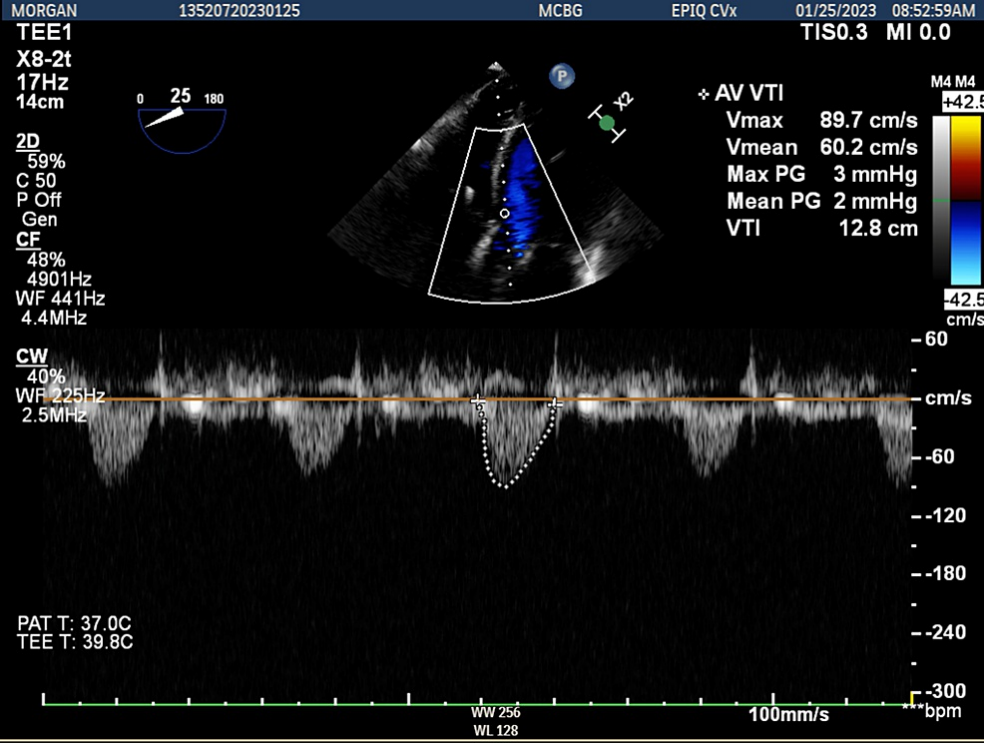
Continuous wave Doppler of the aortic valve post-valvuloplasty
